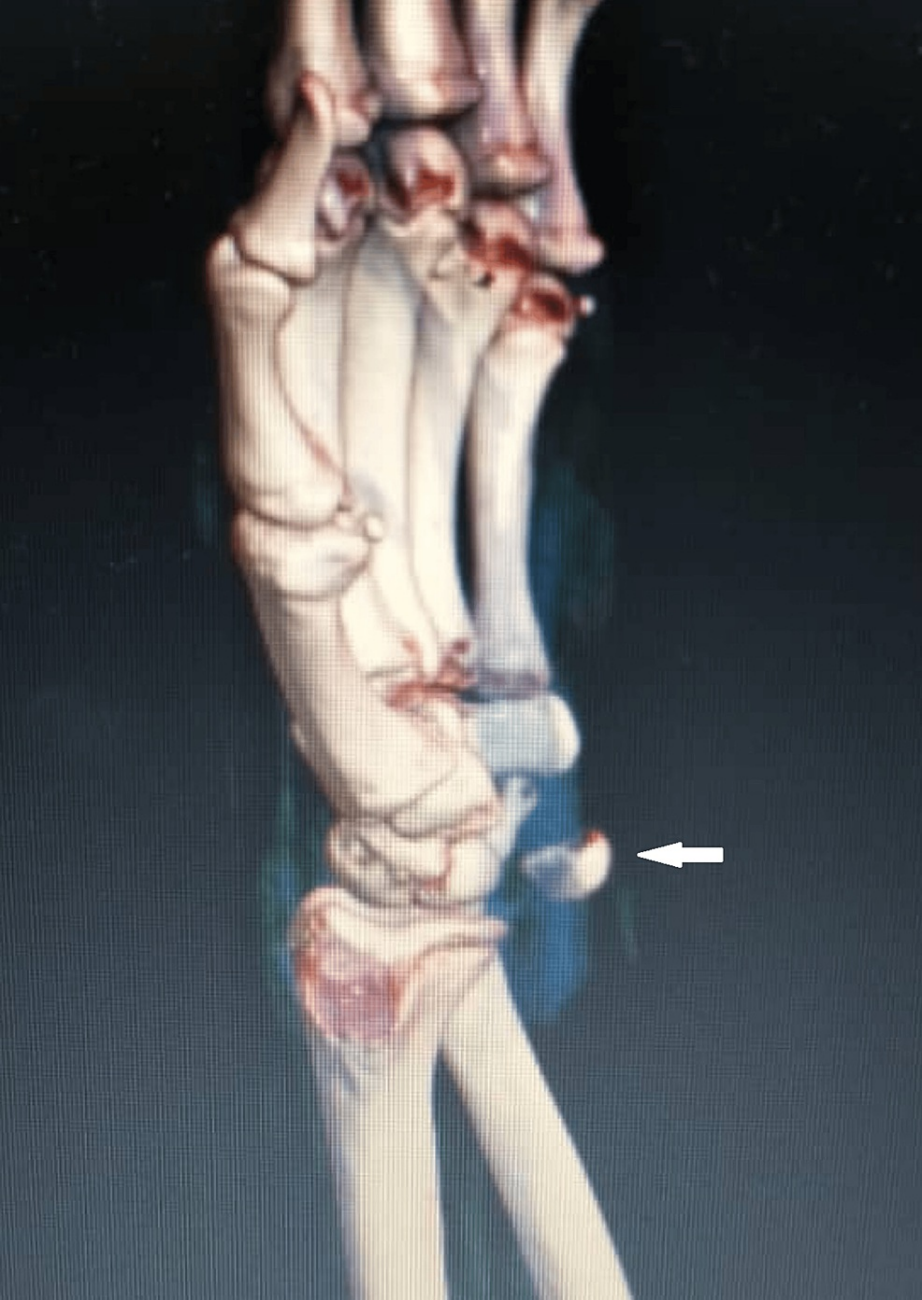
A three-dimensional computed tomography of the left wrist demonstrating the position of the dislocated pisiform bone (white arrow).
